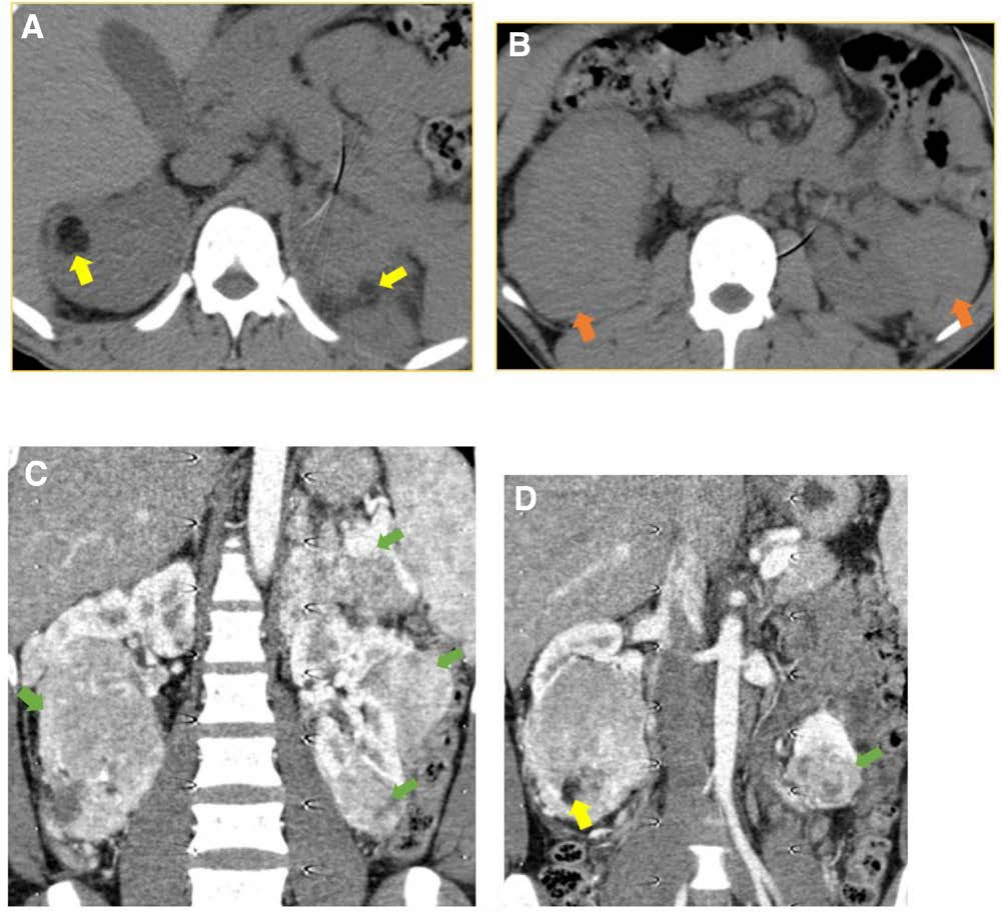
Abdominal CT scan (case-1): The pre contrast study reveals slightly hyperdense, well defined, mildly heterogeneous fat containing (yellow arrows) masses in both kidneys. The masses have variable sizes with ball type, exophytic growth pattern. The post contrast study reveals mildly heterogeneous enhancement (green arrows). Multiple vessels are also seen coursing through the masses (C, D). The findings are consistent with multiple angiomyolipomas (AML) in both kidneys.
